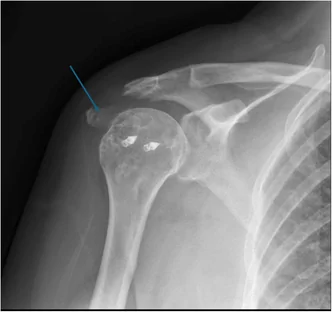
Preoperative radiograph of the right shoulder showing a displaced lateral acromion fracture (blue arrow). There are anchors visible from a prior arthroscopic rotator cuff repair.
